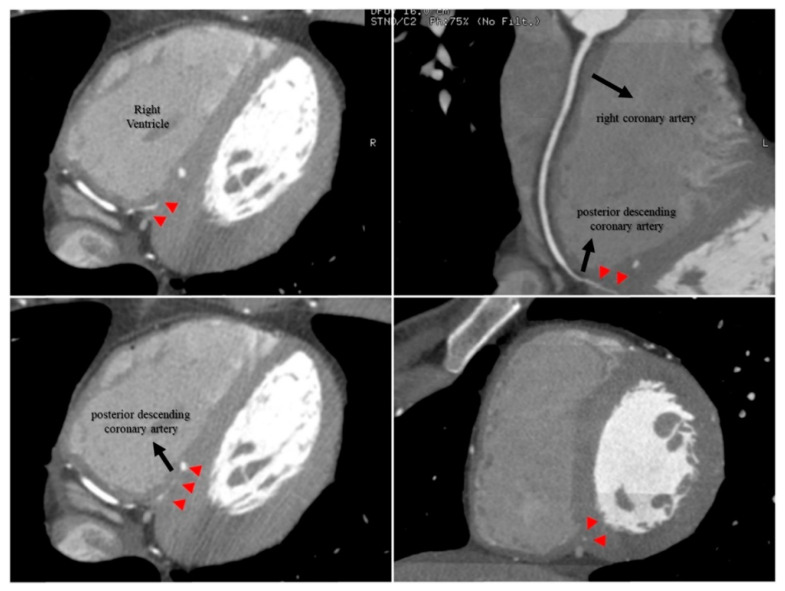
RPD intramyocardial segment shown in CCTA imaging. CCTA showed a short RPD intramyocardial course (red arrowheads) through the posterior interventricular septum instead of traveling its usual course through the posterior interventricular sulcus. CCTA: coronary computed tomography angiography; RPD: right posterior descending coronary artery.
